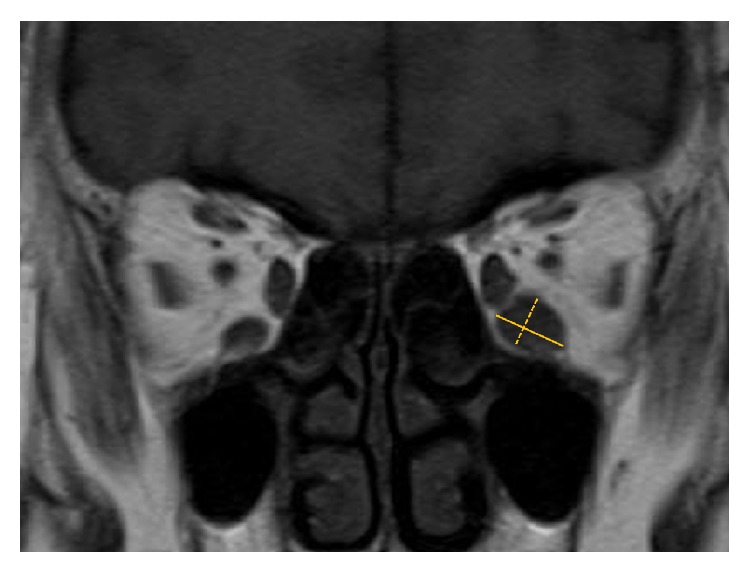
Measurement of the thickness of the inferior rectus muscle (IRM). The major axis of the IRM (solid line) and the minor axis perpendicularly crossing the major axis on the surgical side (dotted line) are measured on the T1-weighted coronal magnetic resonance image showing the largest IRM cross-sectional area.
